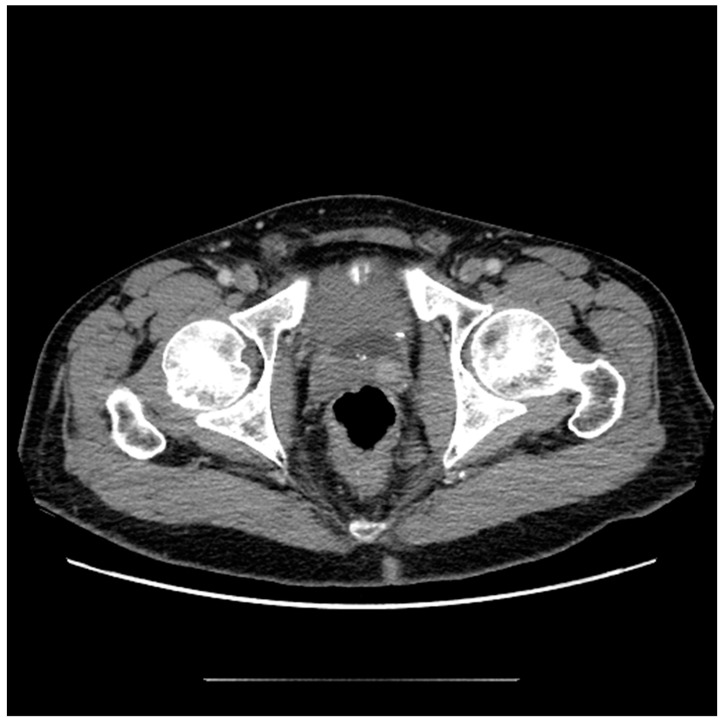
Axial CT image of the pelvis following the administration of intravenous contrast demonstrates focal asymmetric enhancement of the left seminal vesicle, subsequently found to represent prostate cancer at the time of biopsy.
